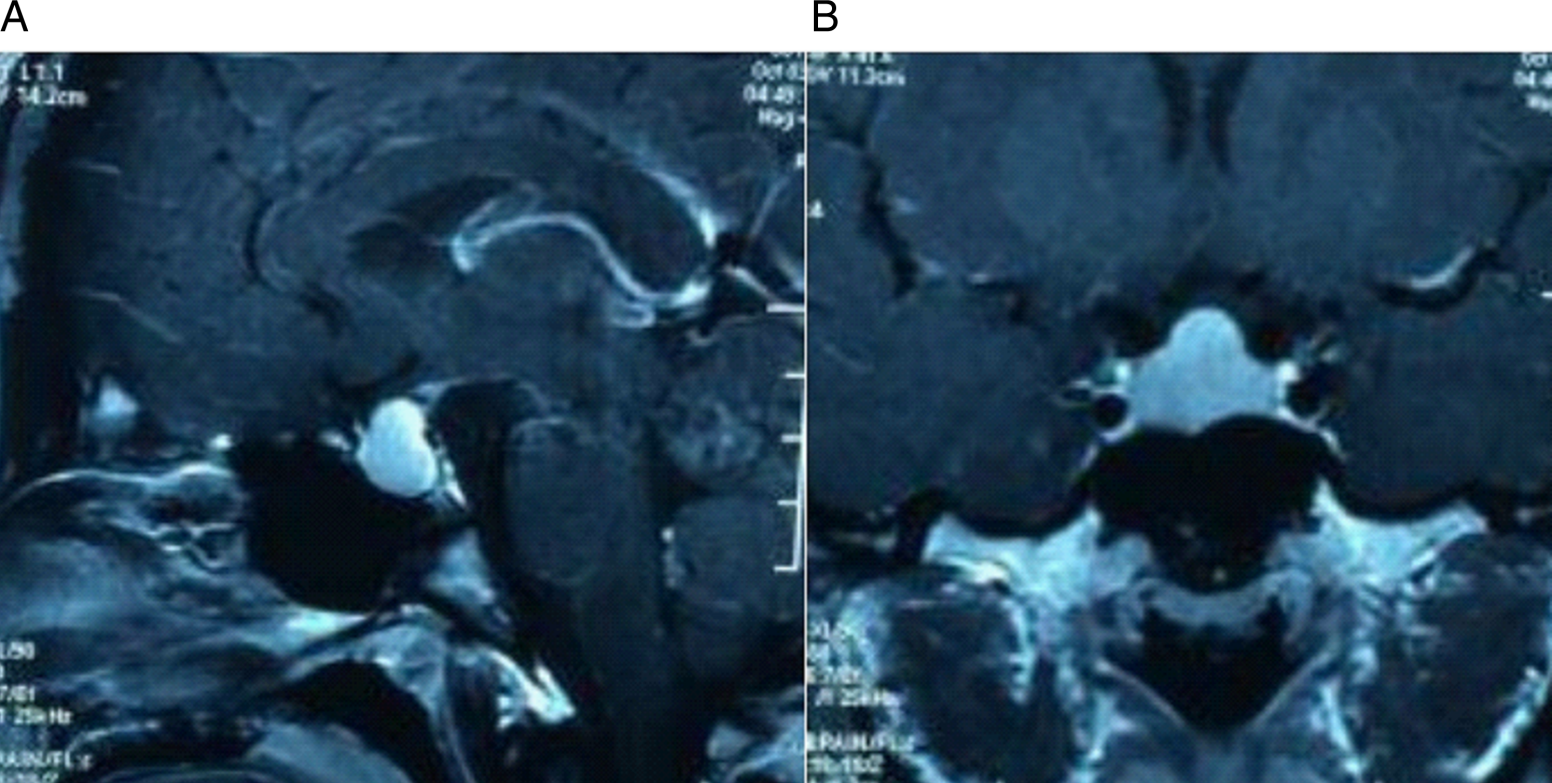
Preoperative MRI. Sagittal (A) and coronal (B) enhanced T1-weighted MRI of a 16-year-old male that presented with gigantism and headache. The images show the tumour extending into the suprasellar cistern.
